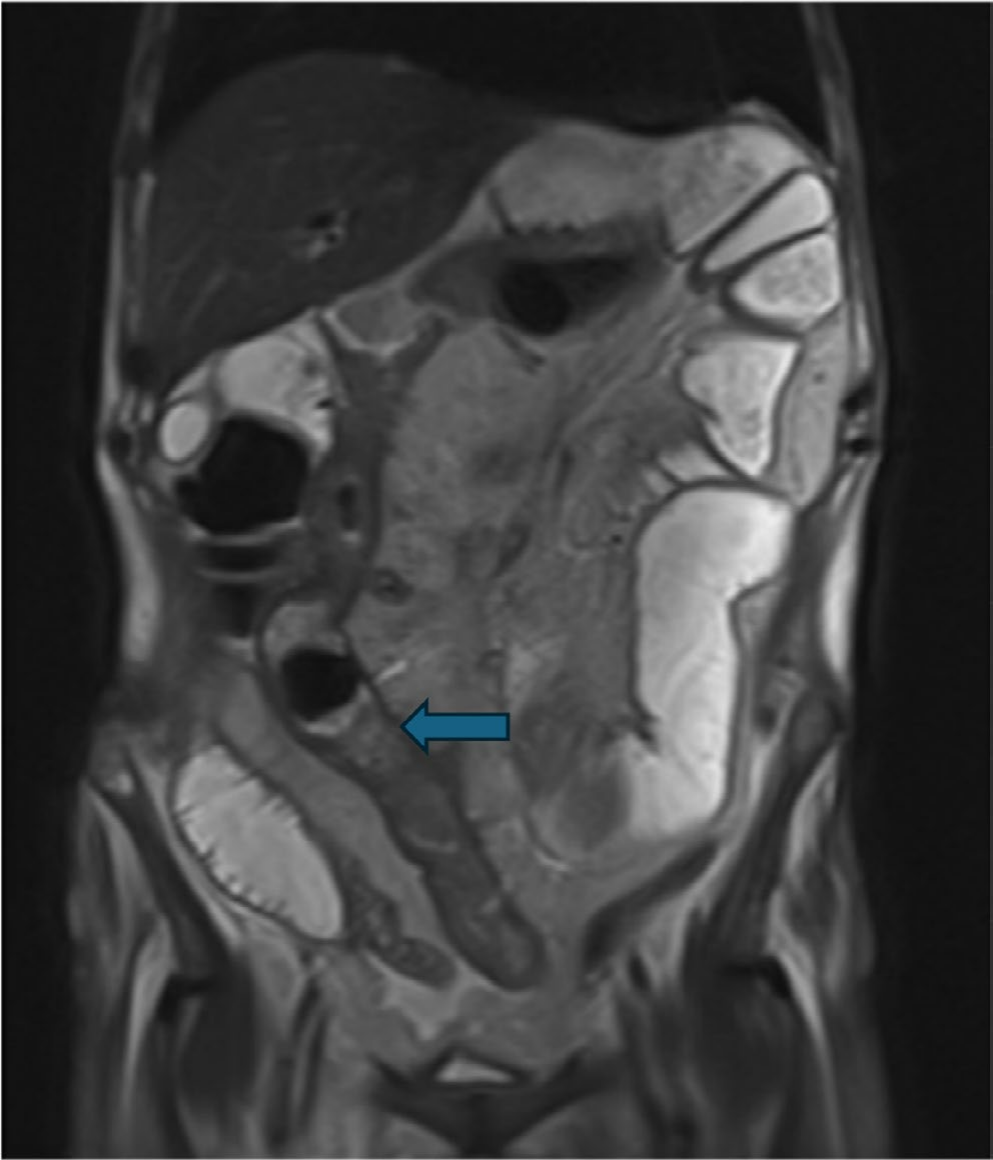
MRI image shows multiple fluid filled and enlarged loops with a hypointense formation (blue arrow) wedged in an ileal loop and causing upstream swelling.
